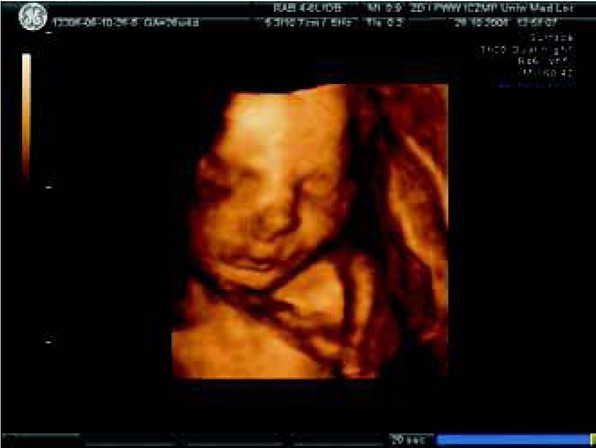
Fetal face in 3D ultrasound at the 26th week of gestation – no dysmorphic feature, however this does not exclude normal or abnormal fetal heart. Ryc. 2. Zarysy twarzy płodu w badaniu USG w prezentacji 3D w 26 tyg. ciąży – nie ma cech dysmorfii, co nie wyklucza obecności wady serca płodu lub prawidłowej budowy serca płodu.
